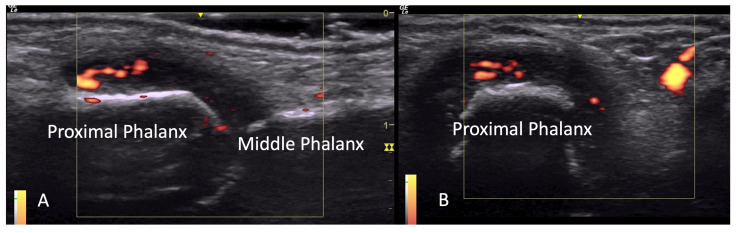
Synovitis seen as hypoechoic intraarticular material, non-compressible, with Doppler signal seen at MCP joint in longitudinal (A) view and transverse (B) view. MCP = metacarpal phalangeal.
